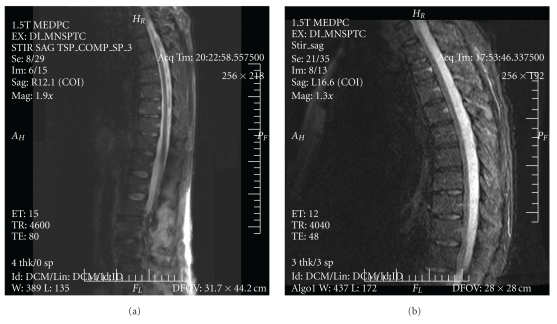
Saggittal thoracolumbar spine MRI with STIR (short T1/tau inversion recovery): day 1 (a) and day 11 (b). Serial MRI images of the thoracic and sacral spinal cord from hospitalization day 1 (a) and day 11 (b) using STIR (short T1/tau inversion recovery) sequences. Panel A shows a 12 × 3 cm multiloculated abscess of the right paraspinal musculature in the L2–L5 region, and subtle enhancement of the L3-L4 transverse processes, consistent with osteomyelitis. There is no epidural abscess. The MRI in (b) shows extensive rim enhancement of the epidural column and severe anterior compression of the spinal cord.
